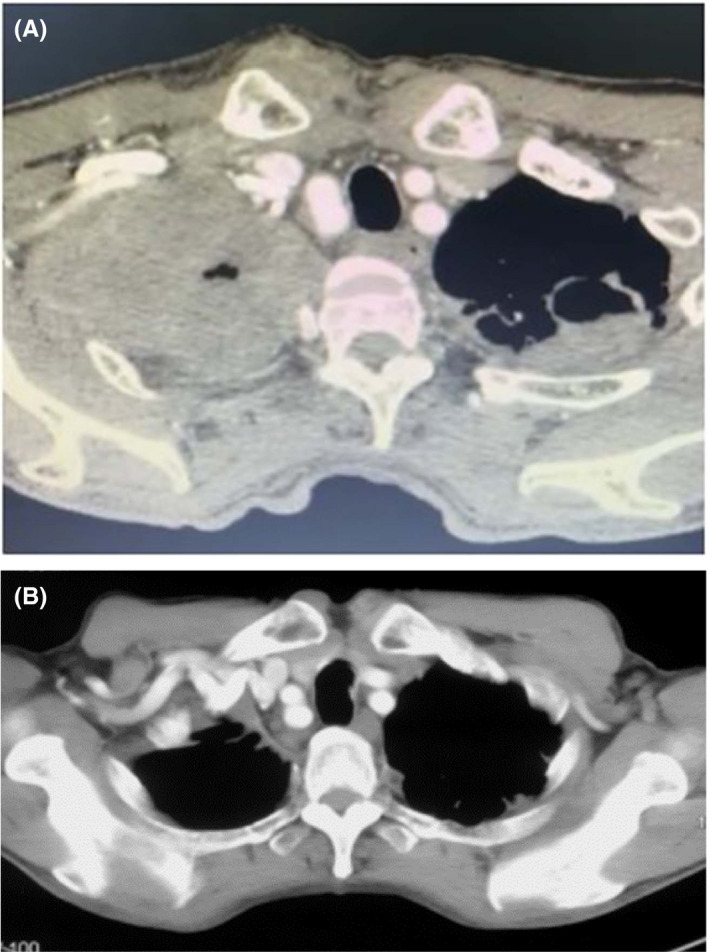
(A) The initial contrast‐enhanced CT thorax in September 2019 showed a large invasive tumor of the right upper lobe with regional bony destruction. (B) A CT thorax in October 2020 showed near complete resolution
